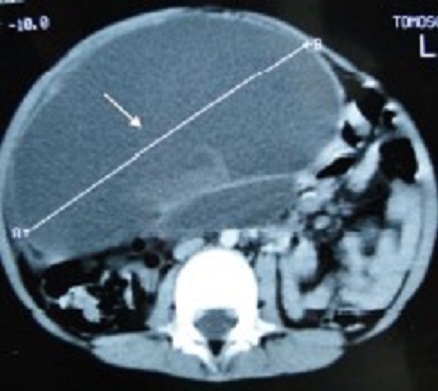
CT scan of abdomen and pelvis showing a large, cystic well defined lesion displacing the bowel anteriorly, it was multiseptated with dense content
